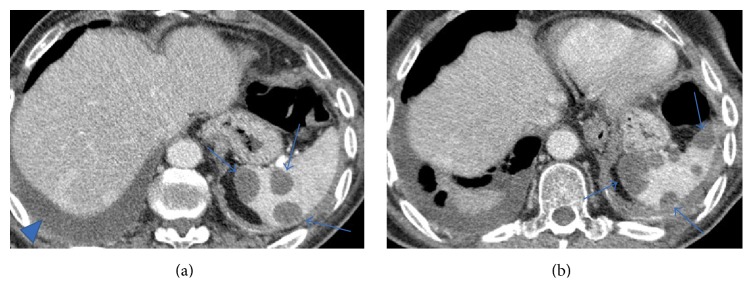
Multislice chest CT. Axial images on mediastinal window at the pulmonary bases (a and b) show increase in size and number of the nodular spleen lesions (arrows). A pleural effusion in the right side is also apparent (arrowhead).
